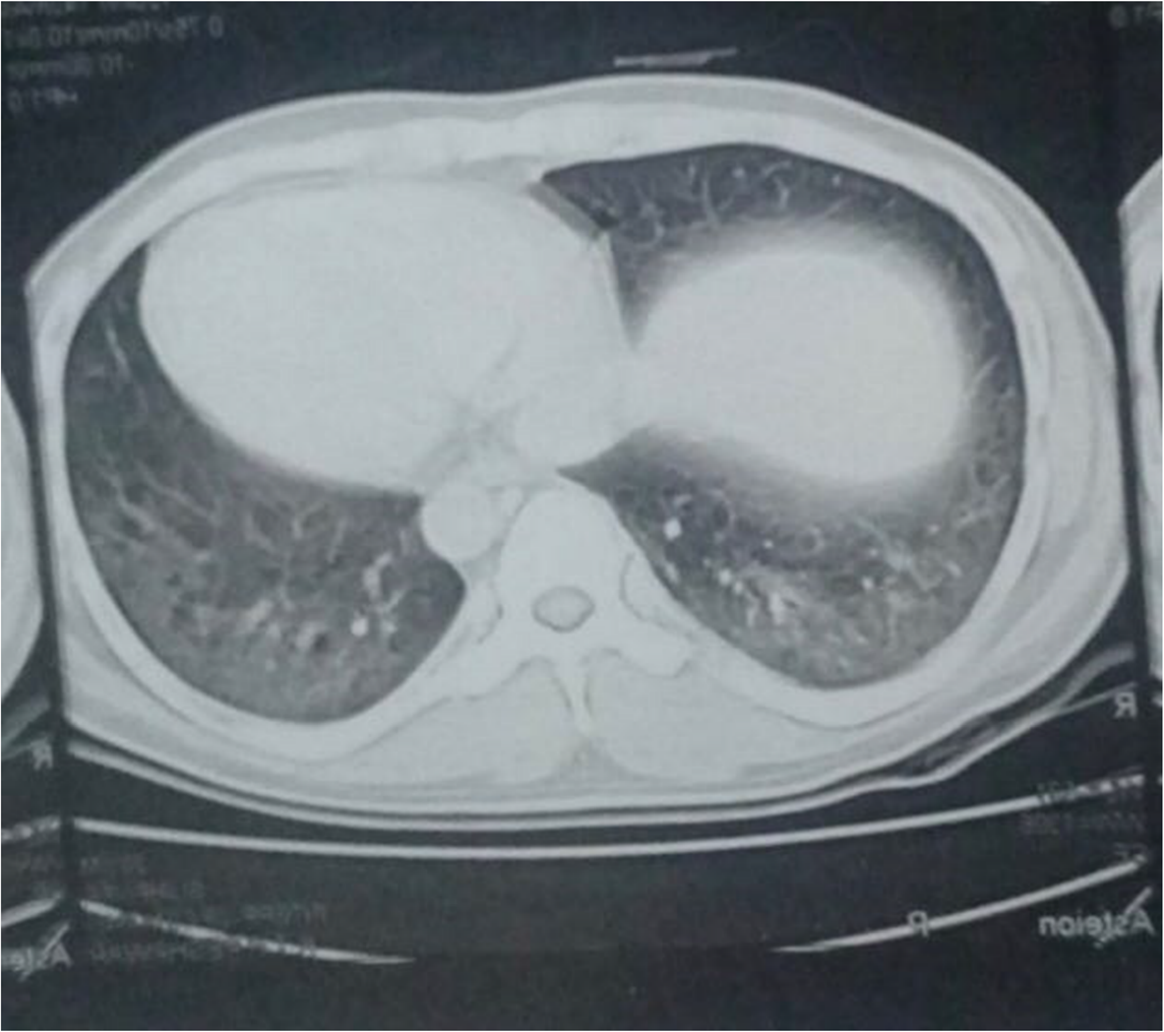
CT scan showing areas of ground glass haze and traction bronchiectasis
